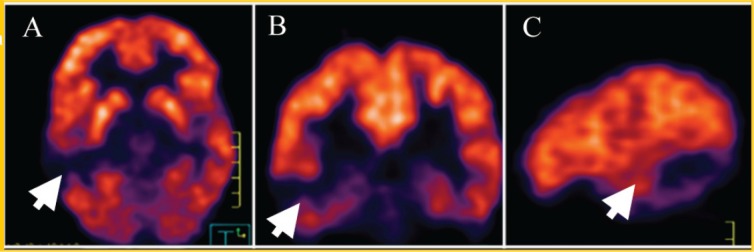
PET (5-FDG) in (A) axial (B) coronal, and (C) sagittal projections that show no uptake in the injury at the right temporal level (white arrow).
